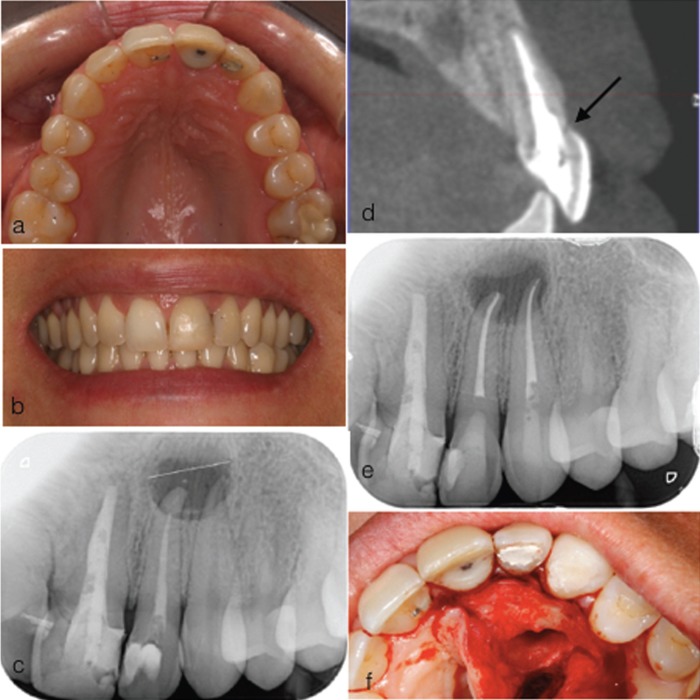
(a) Pre-operative palatal view (b) pre-operative labial view (c) pre-operative radiograph (d) CBCT, arrow showing fracture line (e) root canal treatment of UL2 and UL3 (f) cyst enucleation.
